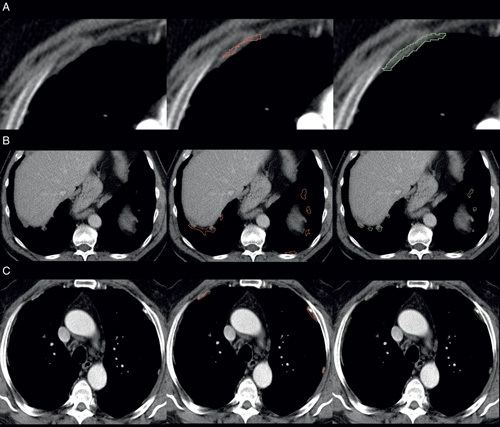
Several examples of adjusted segmentation after inconsistencies were noted. The first column is the CT scans, the second column is the segmentation of the first radiologist, and the third column is the revised segmentation. A, CT scans in the axial plane with contrast; PP only partially segmented. B, CT scans in axial plane without contrast; PPs segmented on lung window, leading to overestimation of the volume. C, CT scans in the axial plane with contrast; missed PP.
